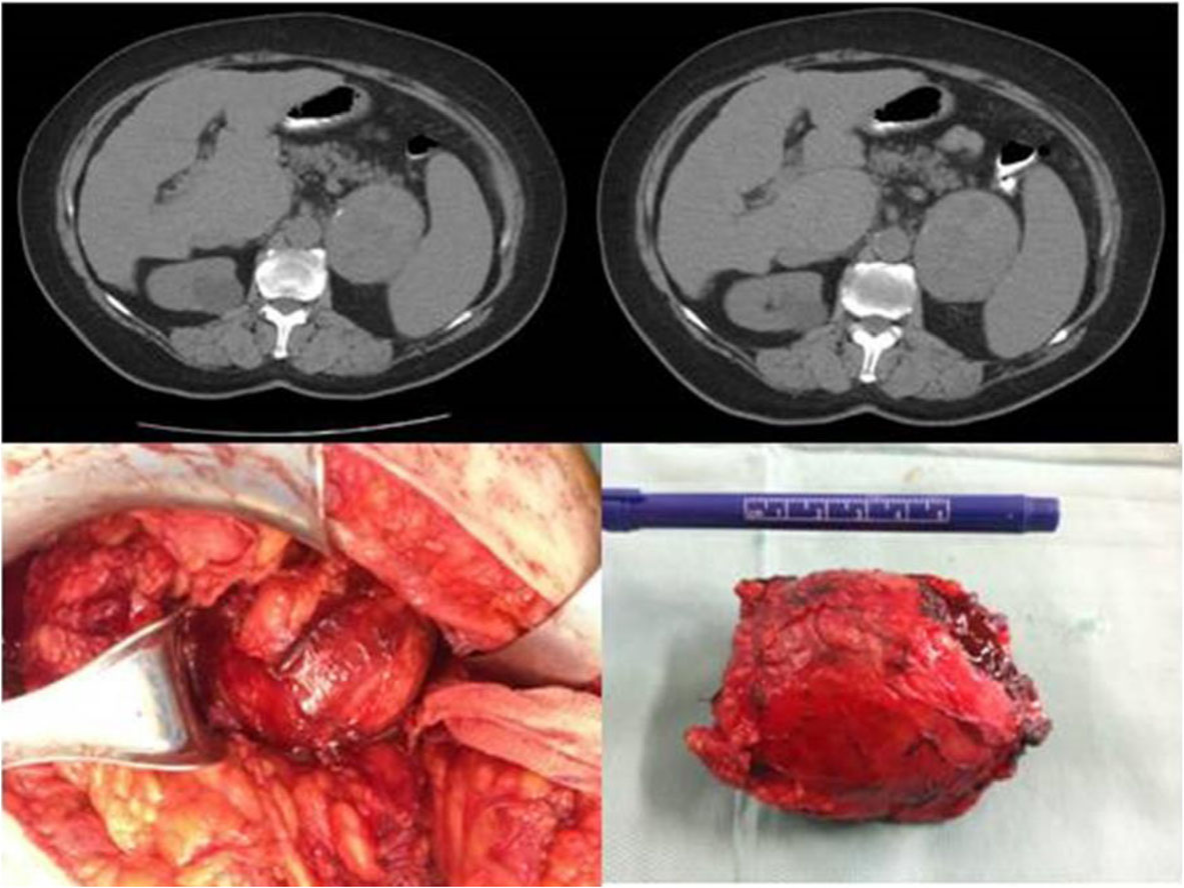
Computed tomography and intraoperative findings of left adrenal pseudocyst
